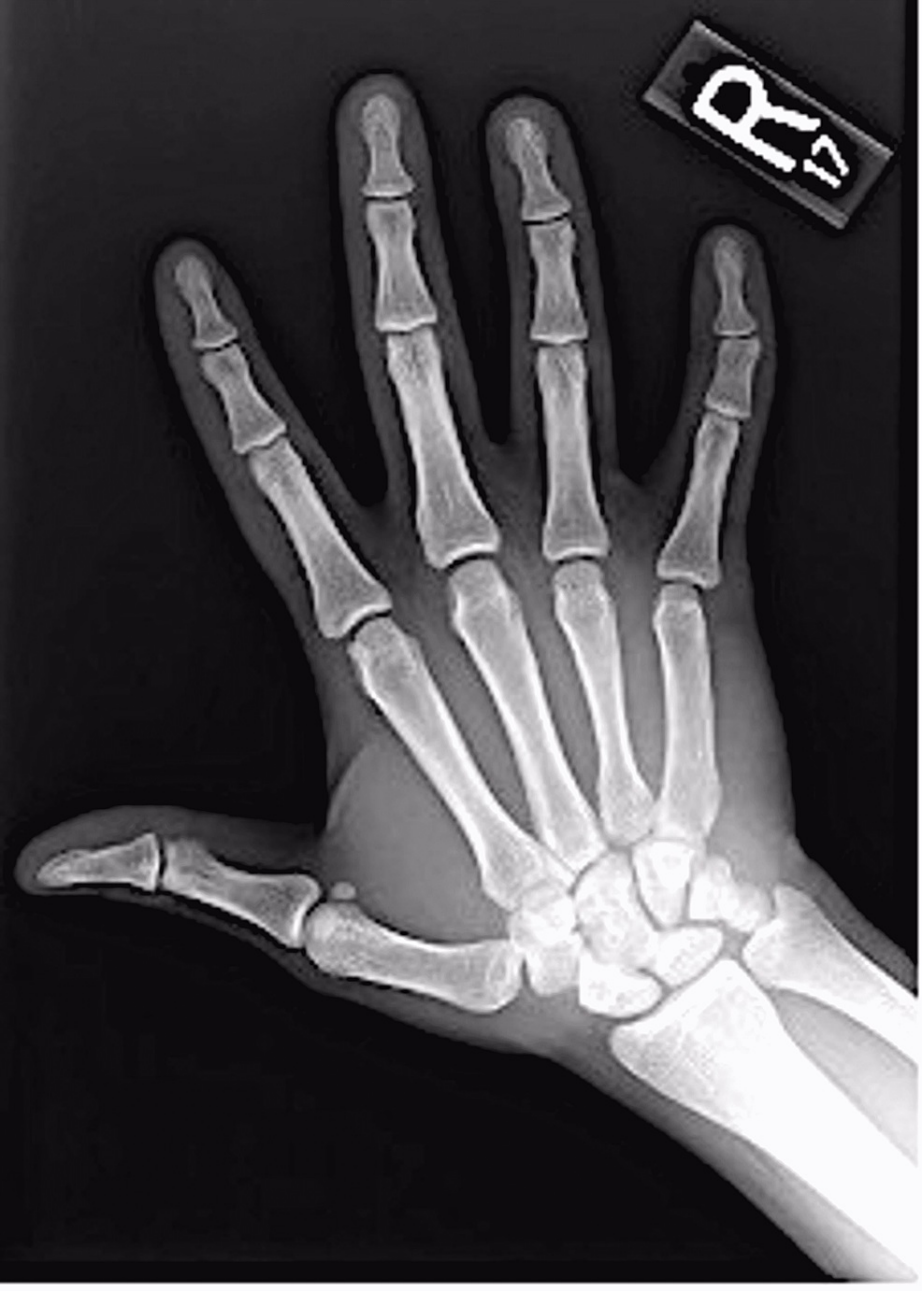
Reticulate acropigmentation of Kitamura presenting with clinodactyly of the right hand on plain film.
